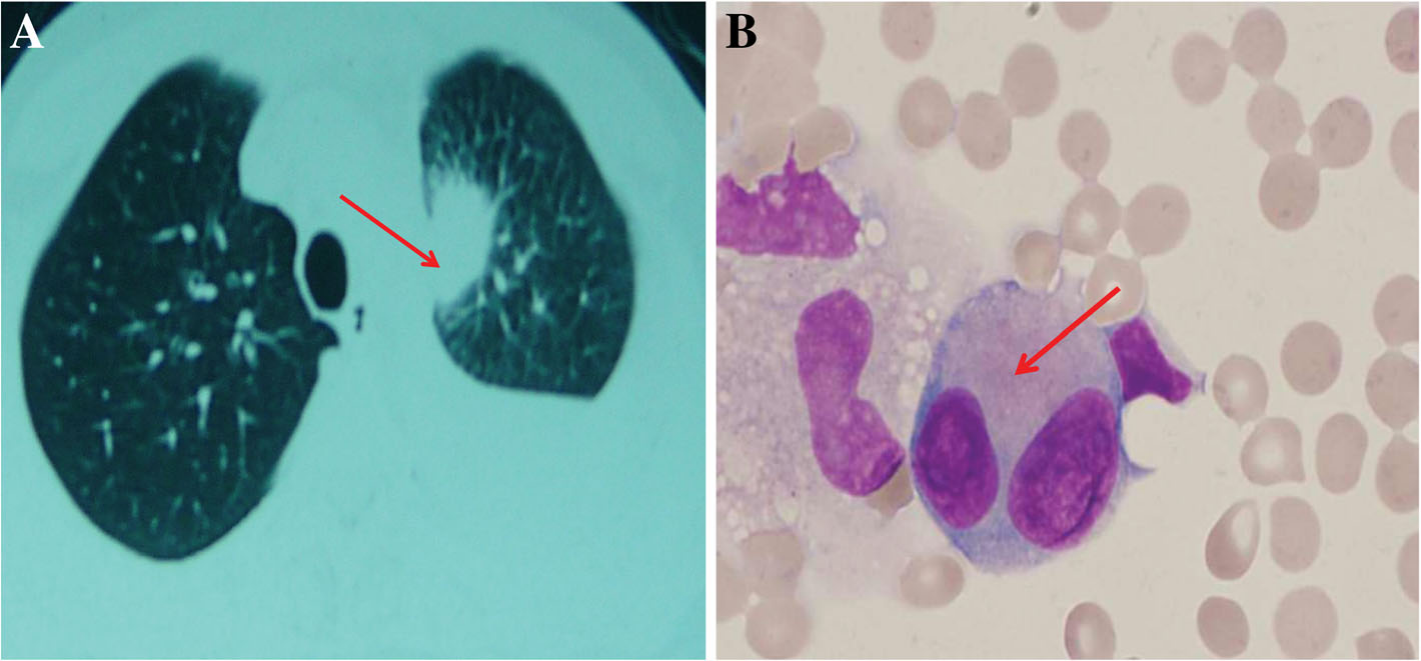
Pulmonary Magnetic Resonance imaging and CSF cytology exams, (Magnification: 1000X) before adjusting the medication based on the NGS. a Left lung placeholder before hospital admission. The red arrow identifies the placeholder. b Cell types in CSF. CSF showed several tumor cells. Lymphocyte cells accounted for 20% of the cell populations, monocytes accounted for 22%, activated monocytes accounted for 40%, and neutrophils accounted for 18%. The red arrow identifies the tumor cell
